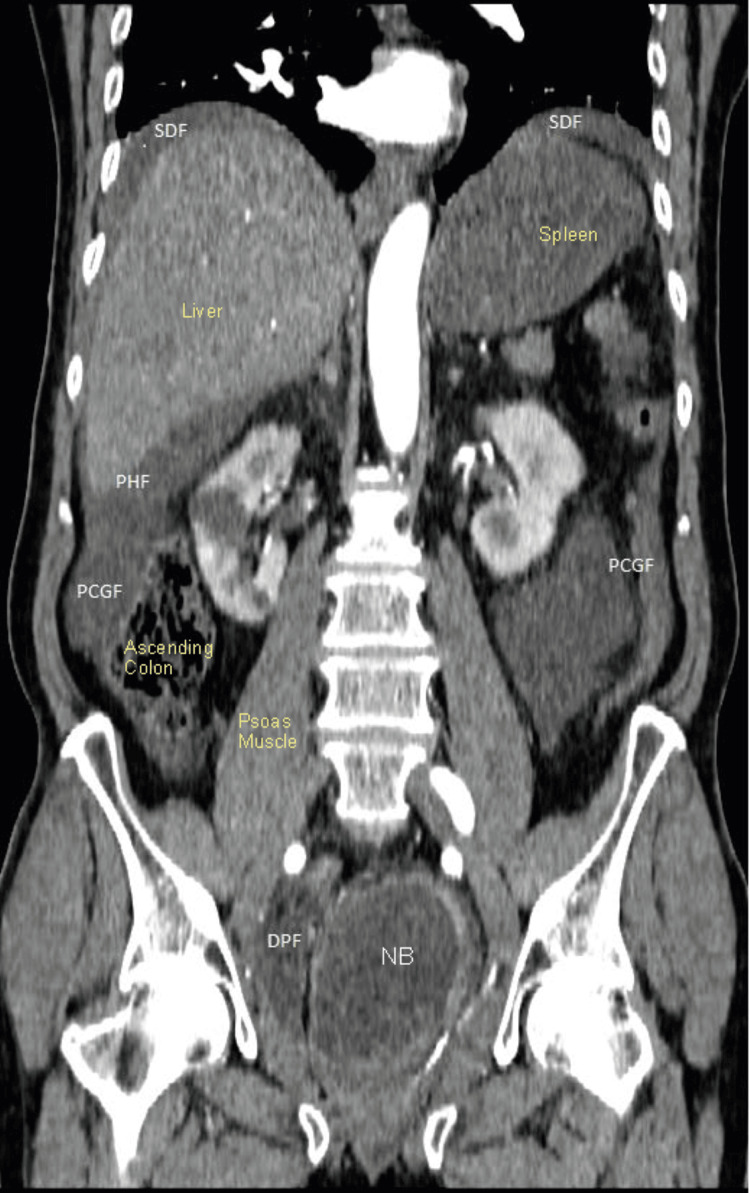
Coronal view of CECT of abdomen and pelvis performed on the day of admission showing moderate-volume free fluid in the deep pelvis, paracolic gutters, perihepatic, and subdiaphragmatic spaces. There was no pneumoperitoneum CECT: Contrast-enhanced computed tomography; SDF: subdiaphragmatic fluid collection; PHF: perihepatic fluid collection; PCGF: paracolic gutter fluid collection; DPF: deep pelvis fluid collection
